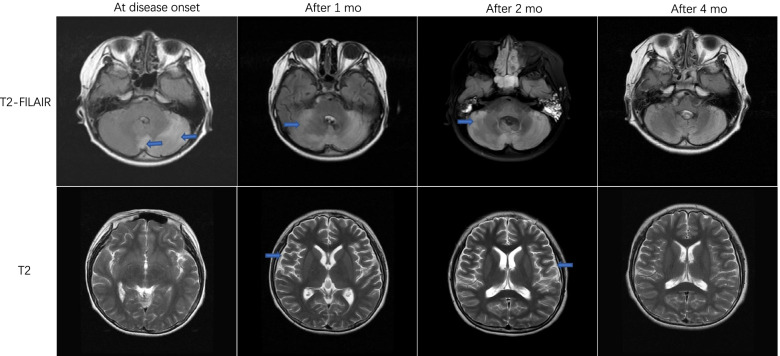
Representative brain magnetic resonance imaging at disease onset, after 1 month,2 months and 4 months in the fulminant encephalitis patient. The lesions of cerebellar hemisphere and cerebellar vermis(arrowhead) were identified in T2/fluid-attenuated inversion recovery (FLAIR) imaging. T2 Signal were shown cerebral parenchymal atrophy(arrowhead) after 1 month of disease and basically recovered after 4 months
